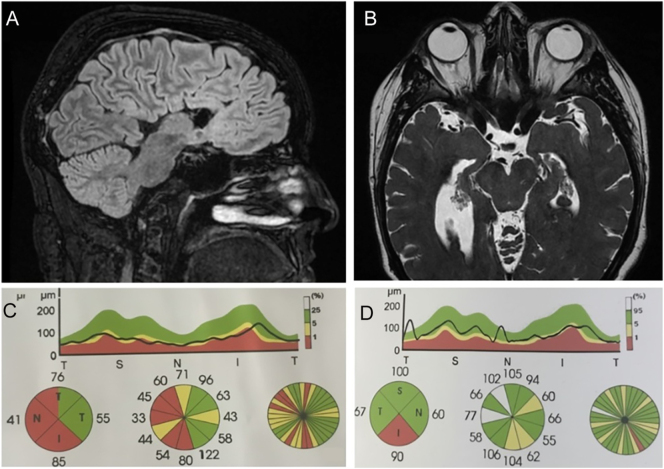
Brain MRI (A and B) and OCT examination (C and D). (A) Complete agenesis of the corpus callosum (as indicated by the arrow). (B) Bilateral hypoplasia of the optic nerves (more evident on the left) with reduced dimensions of the infraorbital and intracranial tracts (as indicated by the arrows). The RNFL OCT examination showed altered values in some sectors: left eye (C) > right eye (D).
